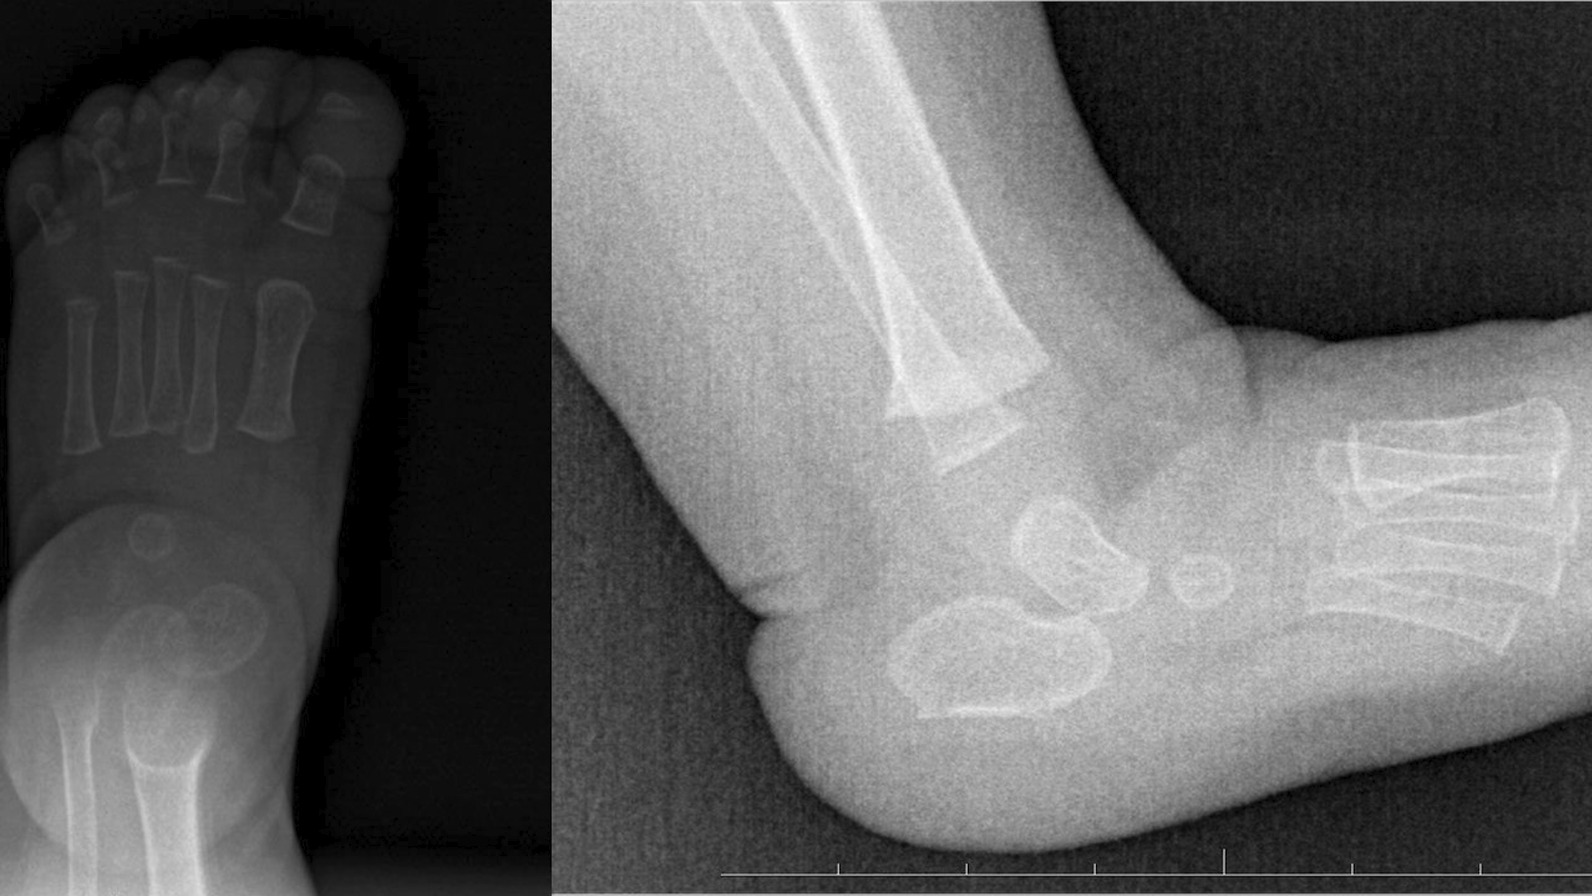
Case 1 AP and L X-ray before surgery (after 5 casts)
